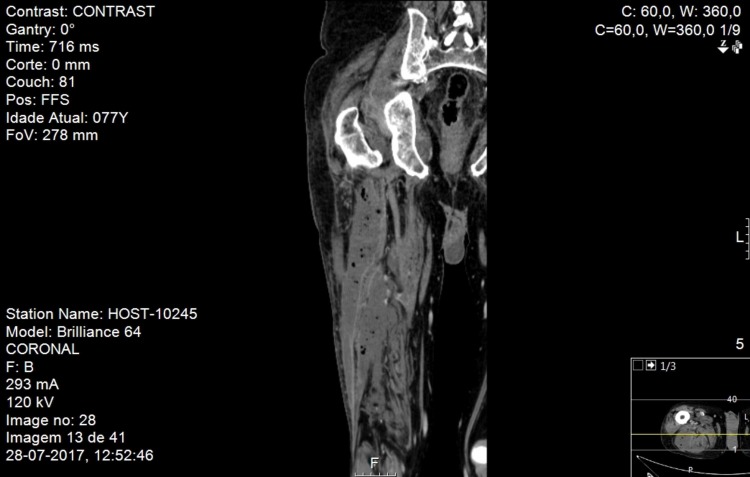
Right lower limb CT scan, frontal plane Right lower limb computerized tomography scan, frontal plane, revealing a gas collection in the region of the right iliacus muscle
